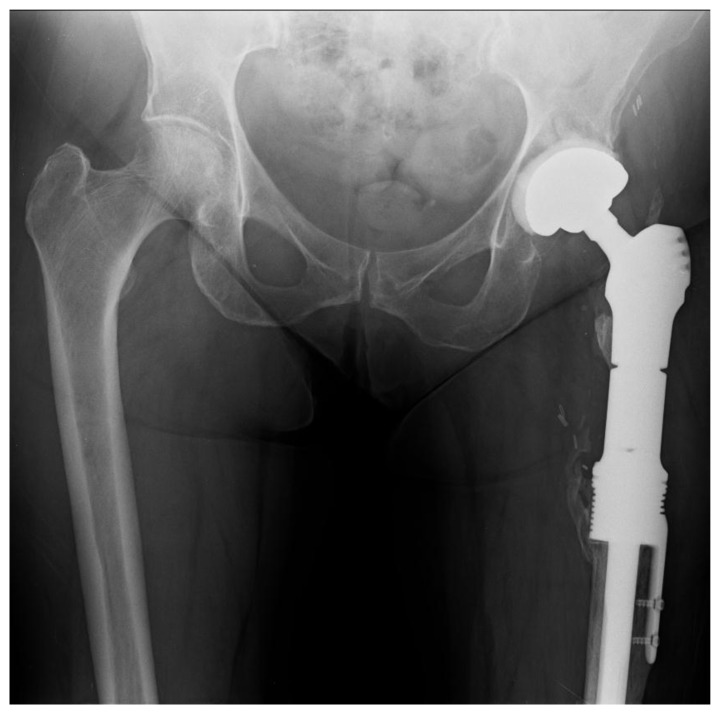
X-ray 3 years following 2nd stage revision with no evidence of loosening and signs of bone integration onto the prosthesis.
